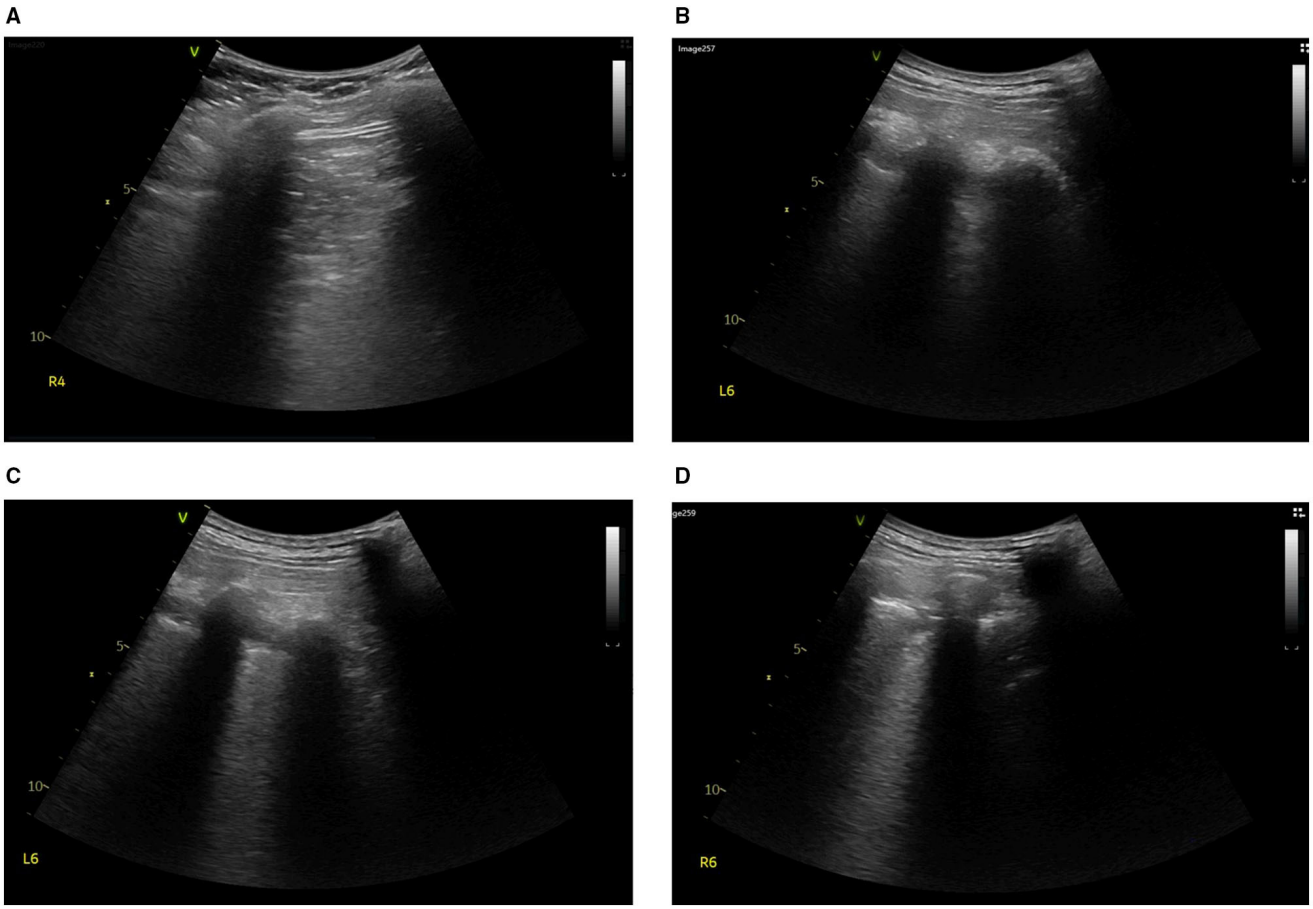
On Day 5, lung ultrasound was performed to find the cause of ventilation defect in the left dorsal lung. Tissue-like signs were found in the region of (A) R4, (B,C) L6, and (D) R6.
